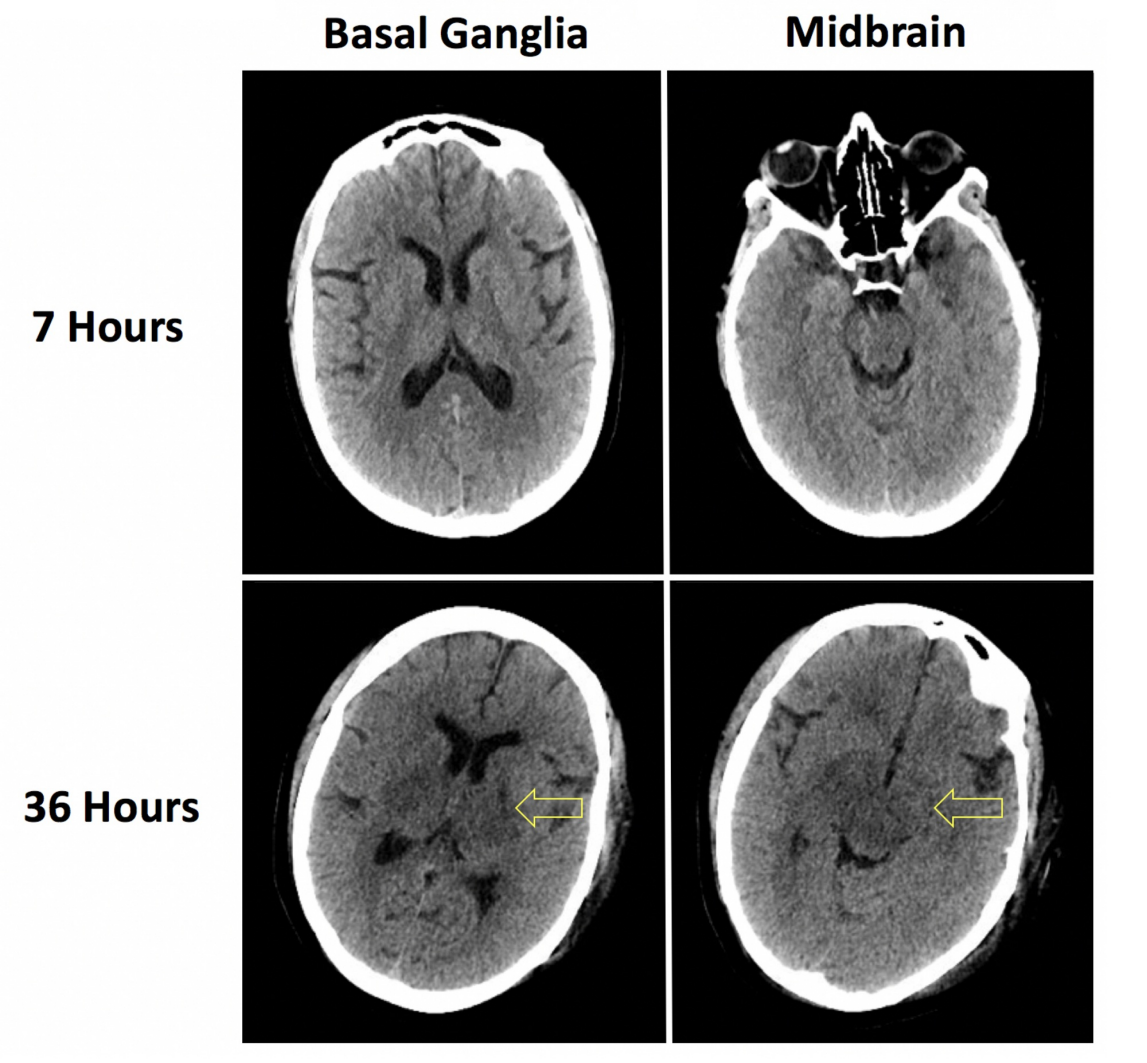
Noncontrast computed tomography imaging Noncontrast head computed tomography imaging acquired 7 hours and 36 hours after presentation revealing interval development of basal ganglia and midbrain hypoattenuation. Although nonspecific, these radiographic findings are consistent with ethylene glycol toxicity.
